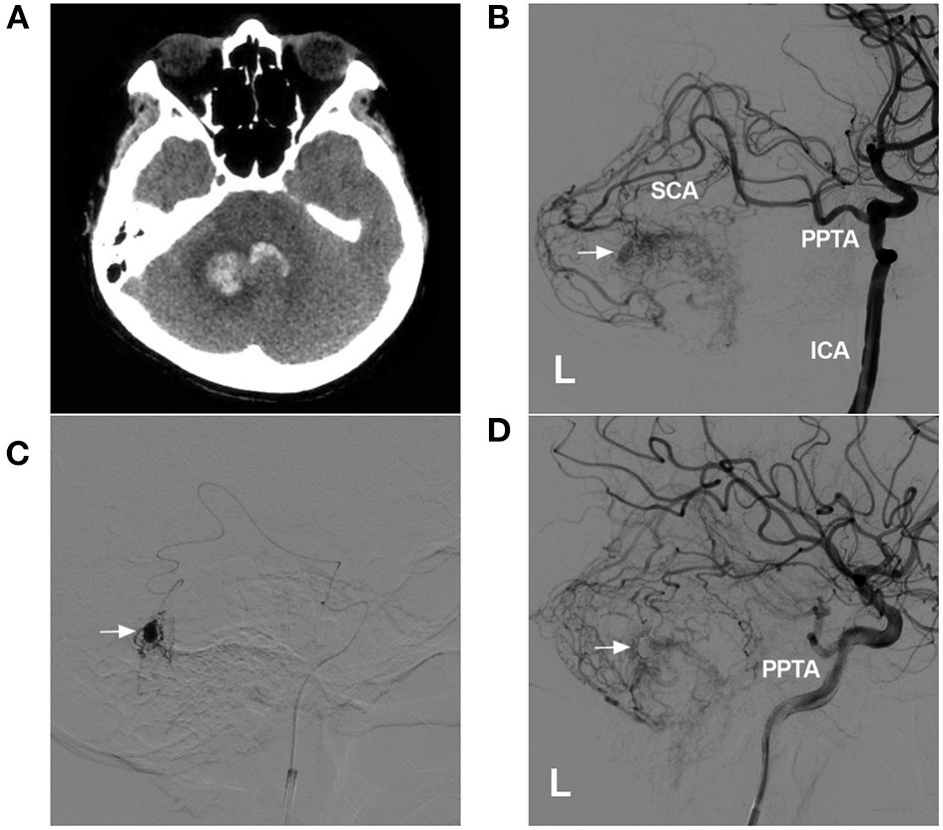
Role of the PPTA as a transarterial path for a BAVM. (A): CTA showing hemorrhage of the cerebellar vermis and fourth ventricle. (B): DSA of the left ICA showing a BAVM supplied by a PPTA via the SCA. An intranidal aneurysm is indicated (arrow). (C): Superselective angiogram of the microcatheter showing the aneurysm (arrow). (D): Postoperative DSA of the left ICA showing that the intranidal aneurysm had been embolized (arrow). BAVM, brain arteriovenous malformation; CT, computed tomography; CTA, computed tomography angiography; DSA, digital subtraction angiography; ICA, internal carotid artery; L, left; SCA, superior cerebellar artery; PPTA, persistent primitive trigeminal artery.
